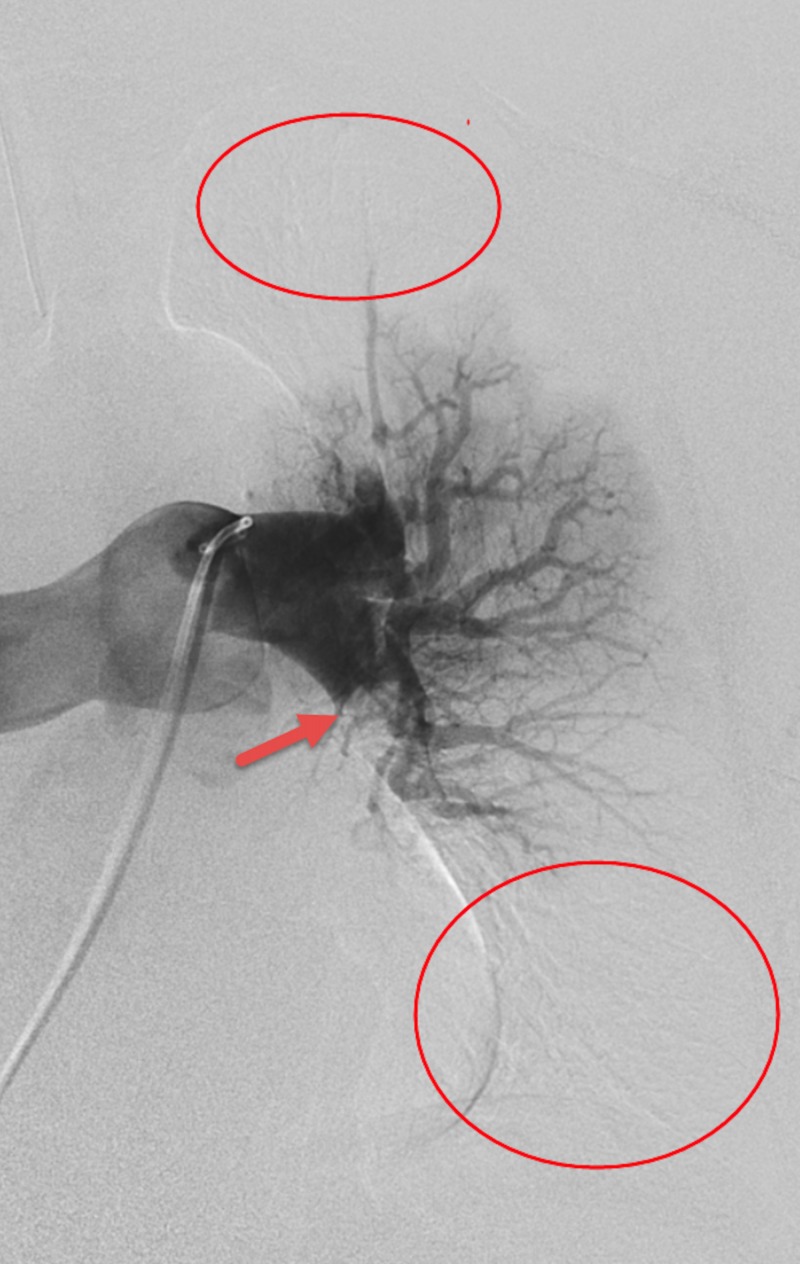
Left Lung Pre-Mechanical Embolectomy CT Pulmonary Angiography Showing Absence of Perfusion to Large Areas of the Upper and Lower Lobes
